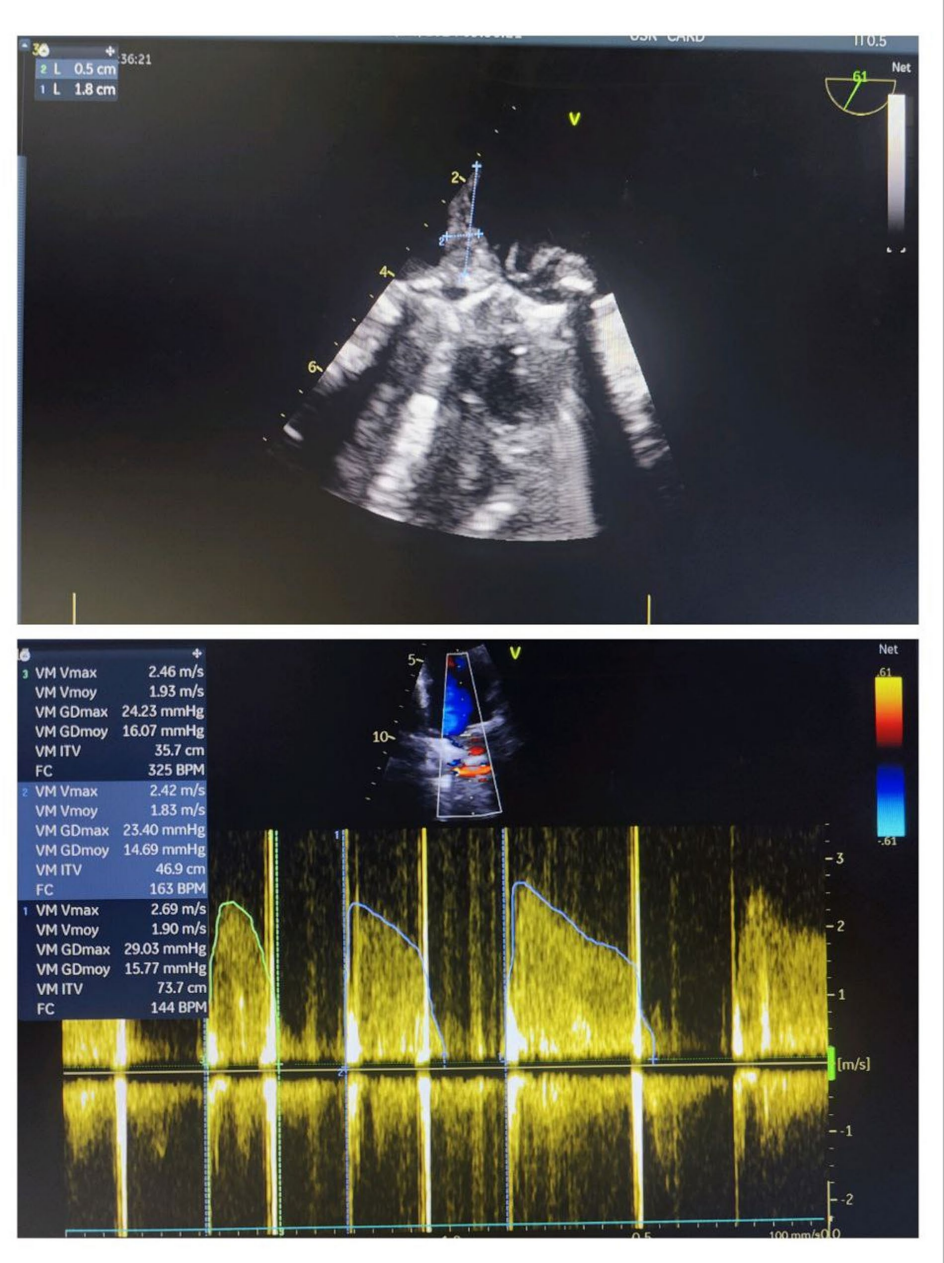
Transesophageal echocardiography (TEE), mid-esophageal mitral valve long-axis view at 61°, demonstrating a vegetation attached to the atrial aspect of the posterior leaflet of the mitral prosthesis, measuring 18 mm by 5 mm, associated with an elevated mean transprosthetic gradient of 15 mmHg
